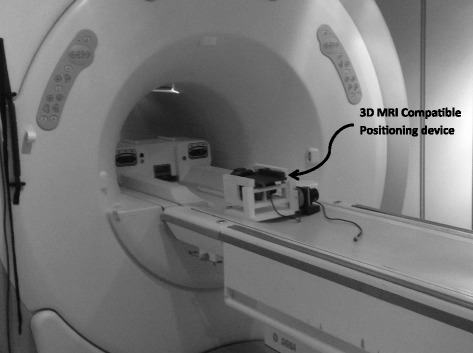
Photo of the 3D positioning device as placed on the table of the GE MRI scanner.
